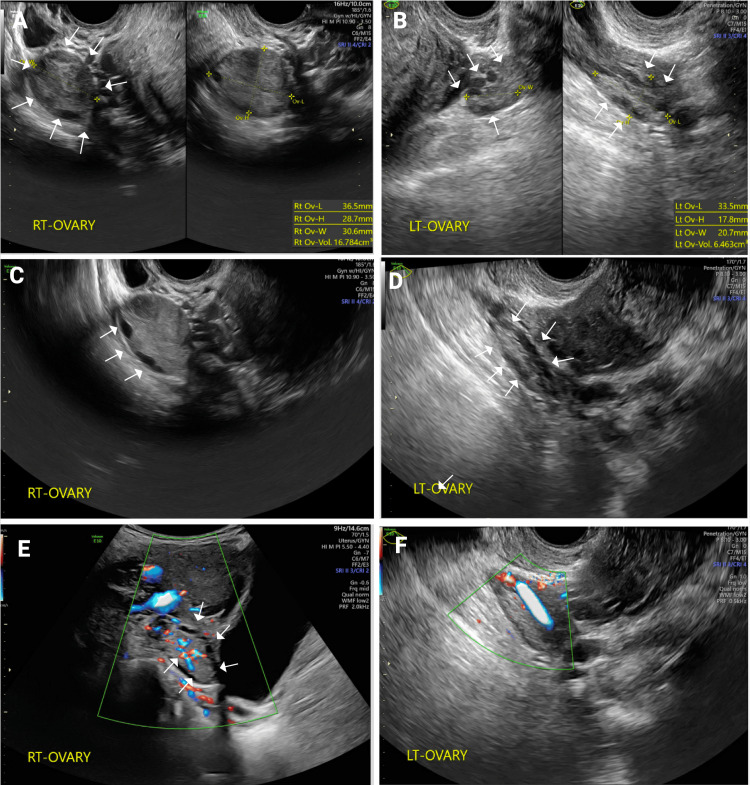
Grayscale and Doppler pelvic ultrasound images obtained three months before the final follow-up visit A, C, and E represent the Rt. ovary, while B, D, and F represent the Lt. ovary. A-D show a good number of antral follicles in both ovaries, indicated by well-circumscribed small black circles, consistent with normal ovarian reserve (ovaries demarcated by white arrows). E and F show Doppler flow with increased vascularity around both ovaries, including a large blood vessel adjacent to the Lt. ovary that could complicate vaginal access to the left ovary. E is a transabdominal image of the right ovary, demonstrating that it is displaced upward by the fibroid and located higher in the pelvis. Scale bars represent approximately 1 mm.
